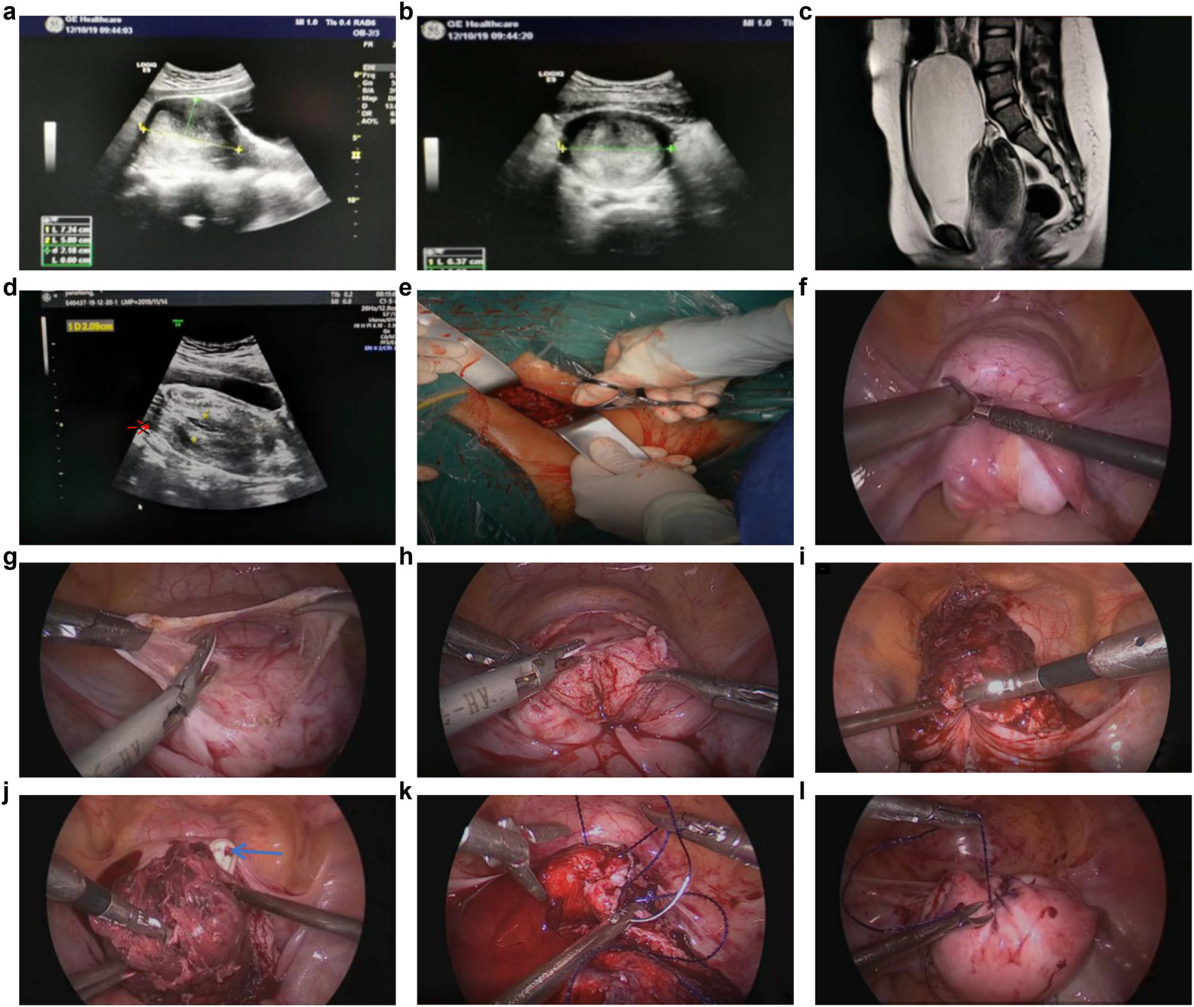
(a and b) Color ultrasound images (sagittal and transverse position) 10 days before the surgery. (c) MRI image after admission. (d) Color ultrasound image after admission (the arrow points to the ovary). (e) Intraoperative vaginal view. (f) Intraoperative laparoscopic view. (g) The uterine serosa was incised. (h) The anterior myometrium and the vagina were incised. (i and j) Uterus after incision (the blue arrow indicates the finger of the vaginal operator). (k) Suturing the vagina and the myometrium. (l) Uterus at the end of suturing.
